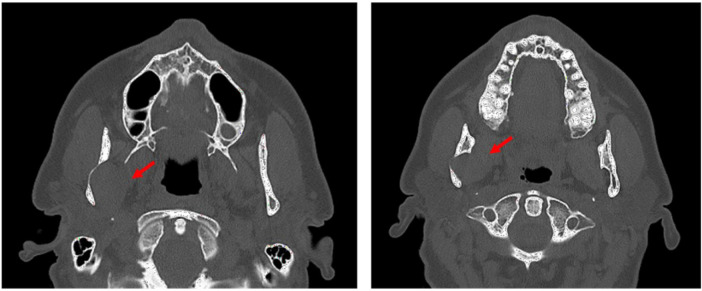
Preoperative CT reveals an ovoid well-defined lesion measuring 2.5 cm located in the right medial compartment of the masticator space, with scalloping over the inner surface of the mandibular ramus, near the mandibular notch and over the Spix foramen. There are no signs of invasion of adjacent anatomical structures.
